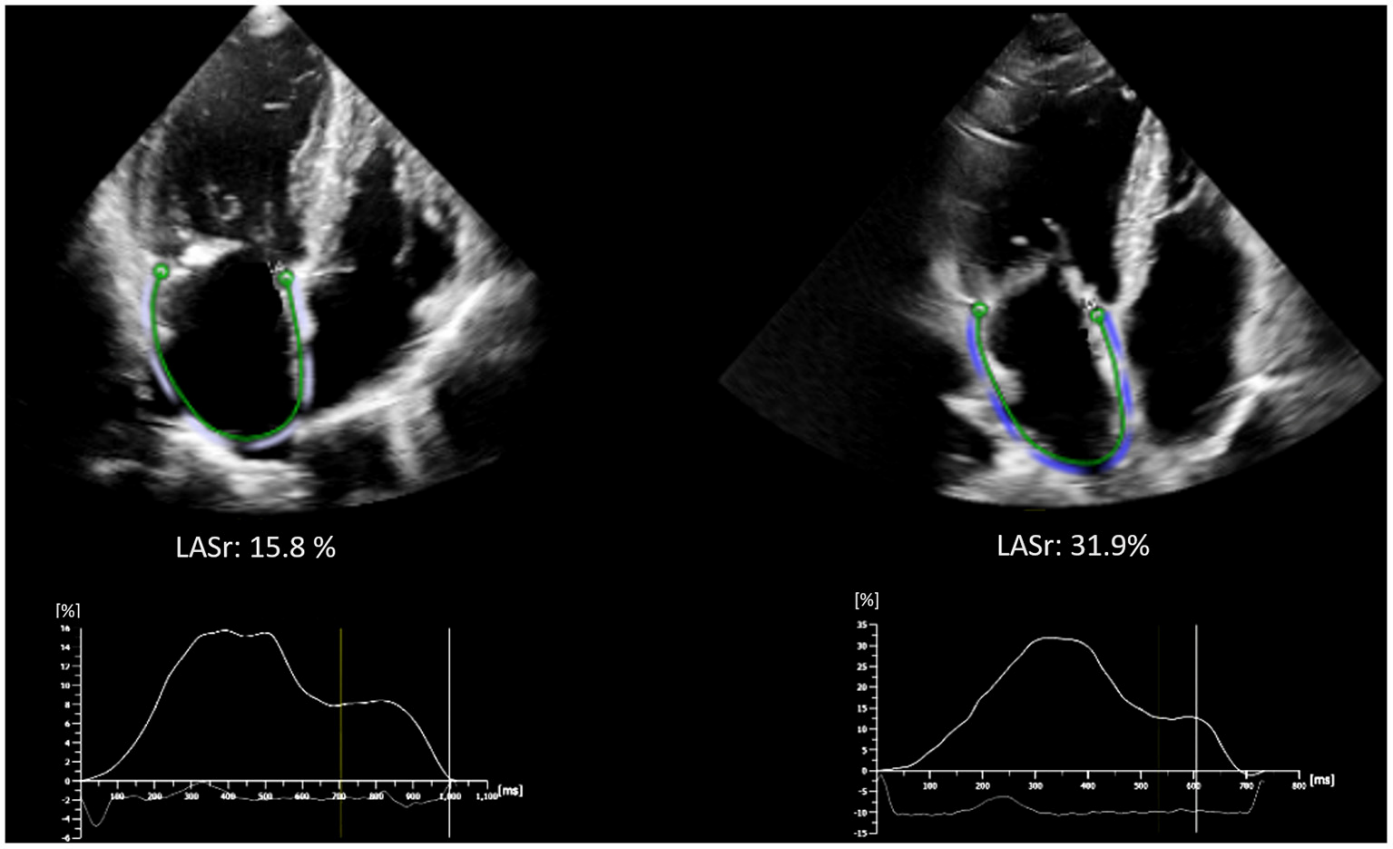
Representative images of LA strain assessment of patients with baseline LASr <20% (left side, LASr = 15.8 %) and ≥ 20% (right side, LASr = 31.9%). Strain curves are depicted below the echocardiographic images. LA, Left atrium; LASr, Left atrial peak reservoir strain.
